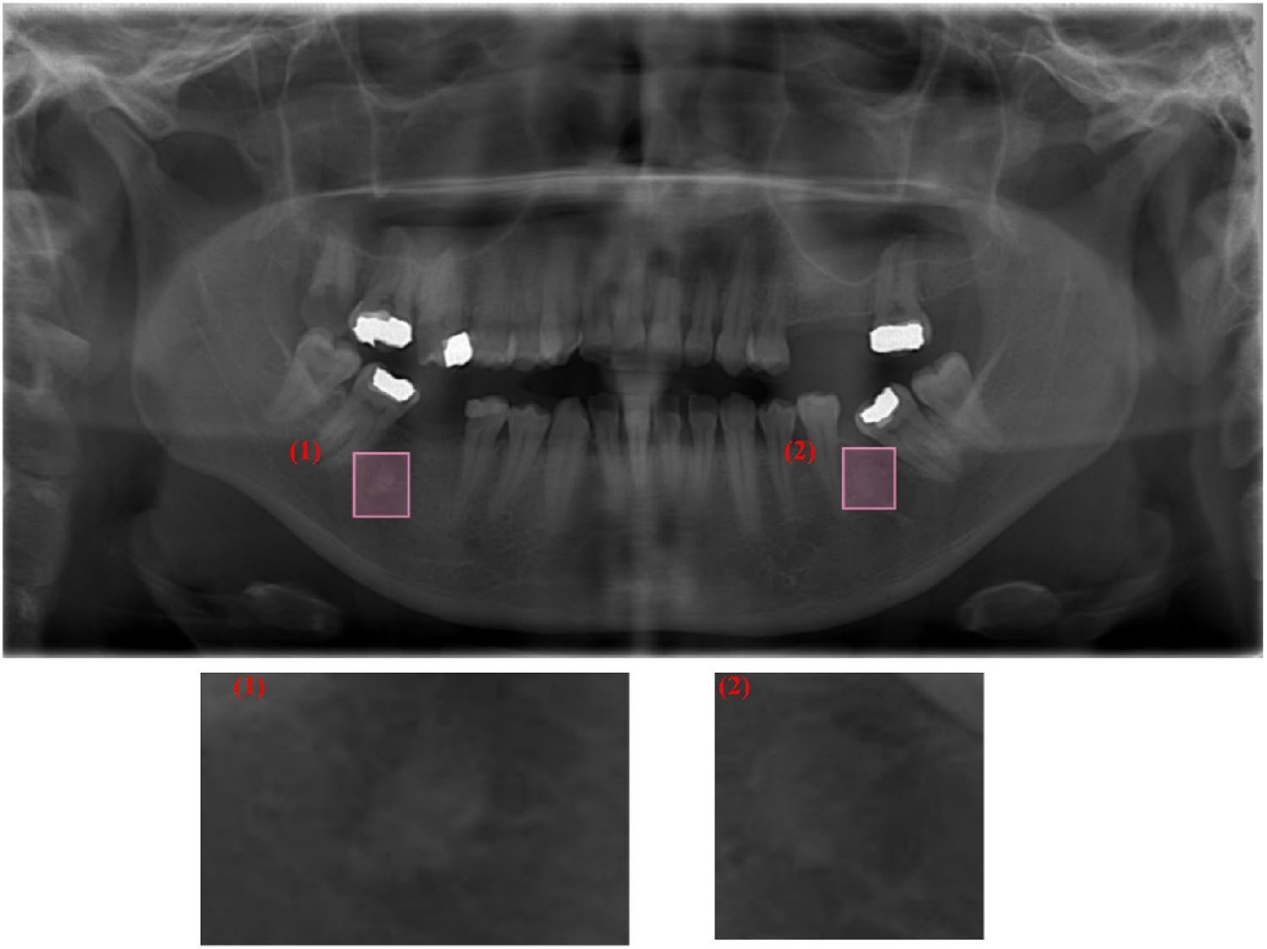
Two annotated IO on low-contrast panoramic radiography and their zooming for close monitoring of pattern changes.
